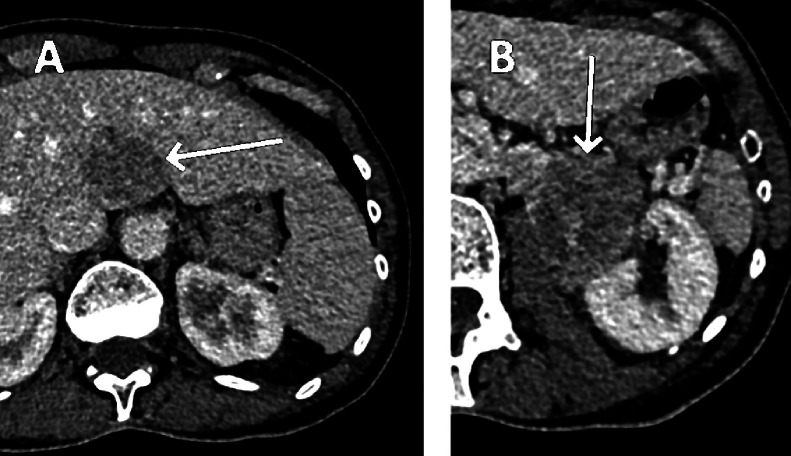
Contrast-enhanced CT of abdomen, acquired during the portal venous phase, showing a liver lesion, later revealed to be a metastasis (A, arrow) and the primary para-renal PEComa (B, arrow).
